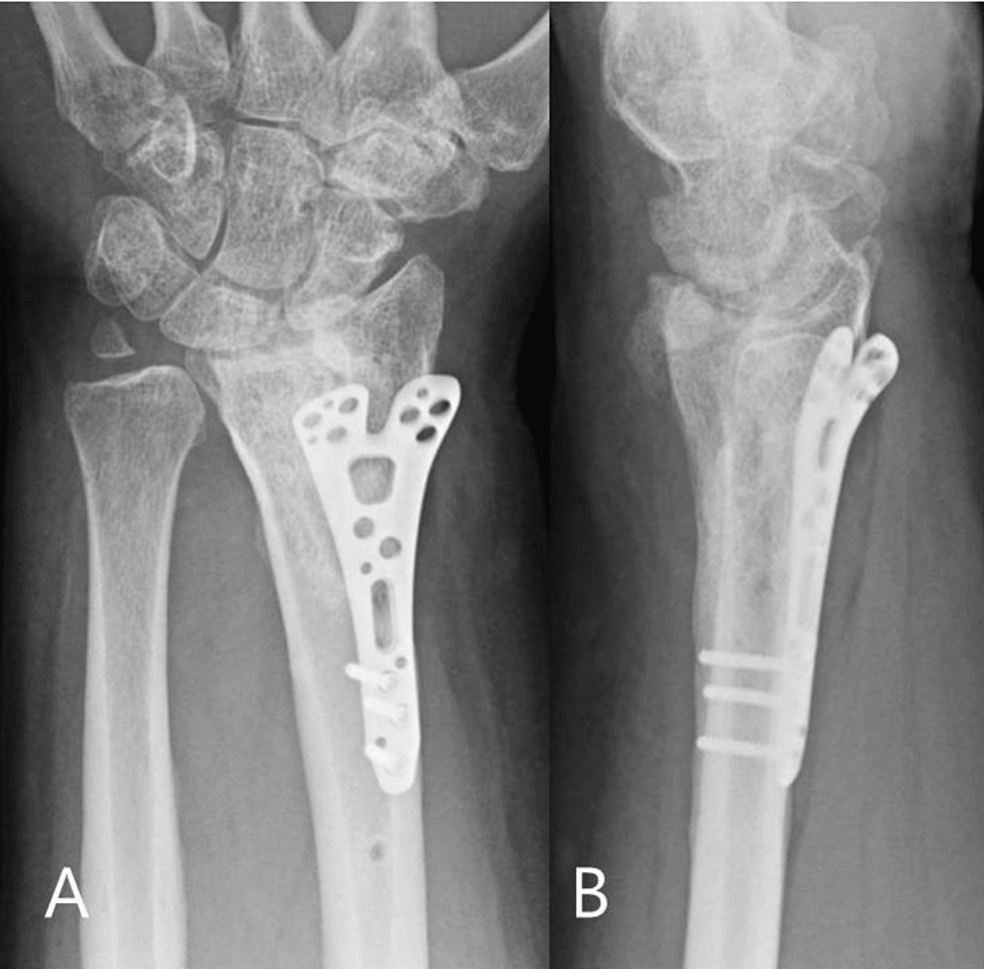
Radiographs at final follow-up A: Showing the restoration of the radial inclination and radial height and the consolidation of the fracture. B: Showing consolidation of the fracture and a small degree of residual dorsal tilt of the distal radius. This was due to the need for early removal of the external fixator as the pin sites became contaminated.
